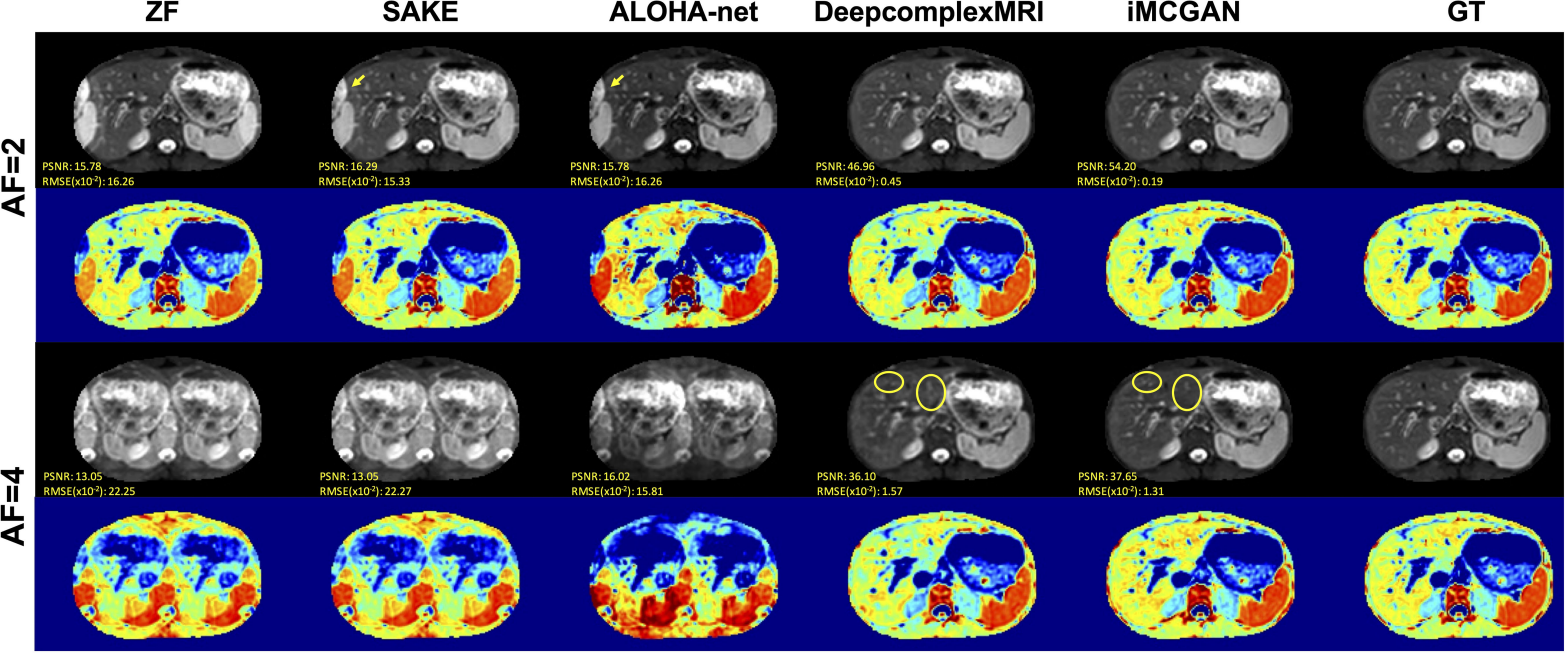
The visual comparison between several advanced DWI (b = 800 s/mm^2^) reconstruction methods under different AFs. The first and third row shows the reconstructed DWI with corresponding PSNR and SSIM values, and the second and fourth row shows the corresponding ADC maps.
